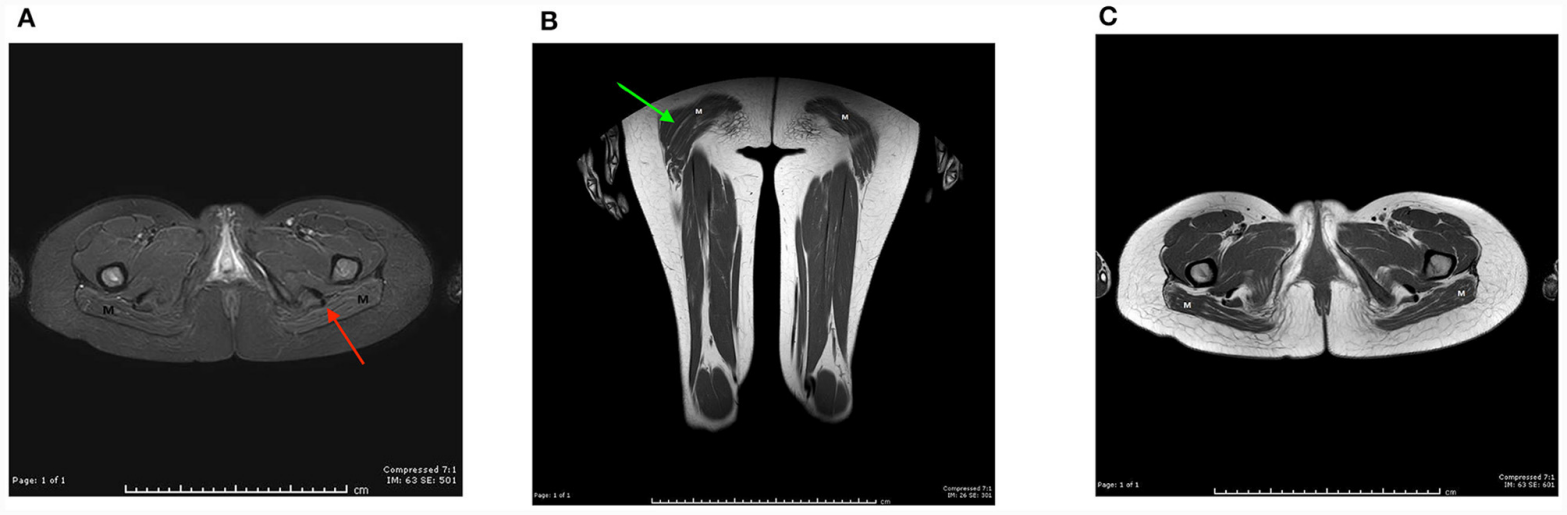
MRI of the pelvis, thighs, upper limbs, and neck was performed on proband 1 using a 1.5T scanner (Toshiba, USA). Axial and coronal T1 and STIR sequences were obtained using a sense neuro vascular coil for the neck, and a multi coil for the rest. Axial T1 image at the level of the pelvic floor (A), and coronal T1 image of the thigh, (B) show symmetrical fat replacement of both gluteus maximus muscles (M), red and green arrows, respectively. The pelvic and thigh muscles bilaterally appear uninvolved. (C) Axial STIR images show suppression of the intramuscular fat (M).
